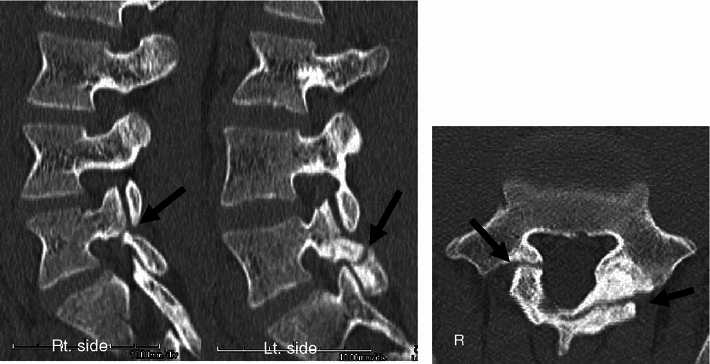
CT images show a pars defect (spondylolysis) on the right side and laminolysis on the left side
